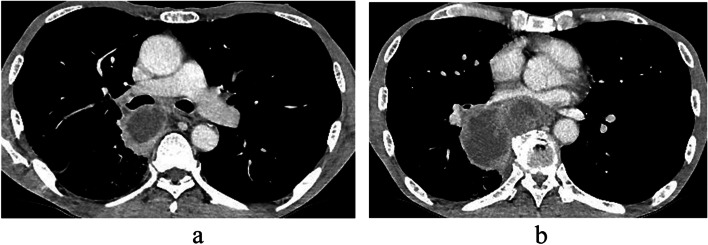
Preoperative computed tomography images. The tumor was 73 mm in diameter and located in the right hilum of the lung (a). It compressed the left atrium of the heart. It appeared to invade the esophagus and a vertebral body (b)
